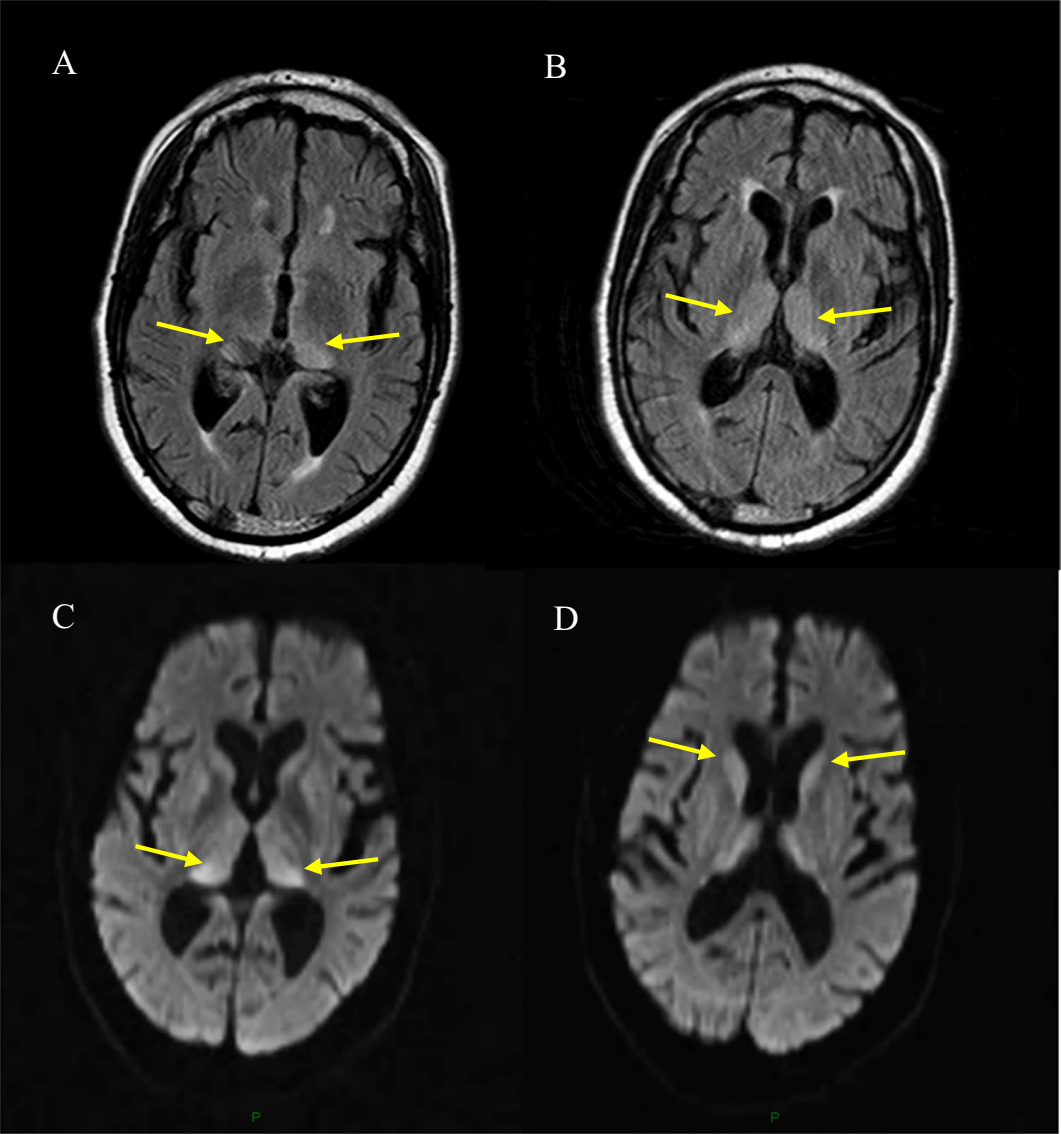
Positive pulvinar sign in definite MV2 sCJD patient. Axial FLAIR sequences (A+B) showing positive pulvinar sign (A) (arrows) with (B) associated dorsomedial thalamic high signal (arrows). DWI sequences (C+D) demonstrating more conspicuous pulvinar high signal (arrows) with (D) associated dorsomedial thalamic and bilateral caudate head high signal (arrows)
